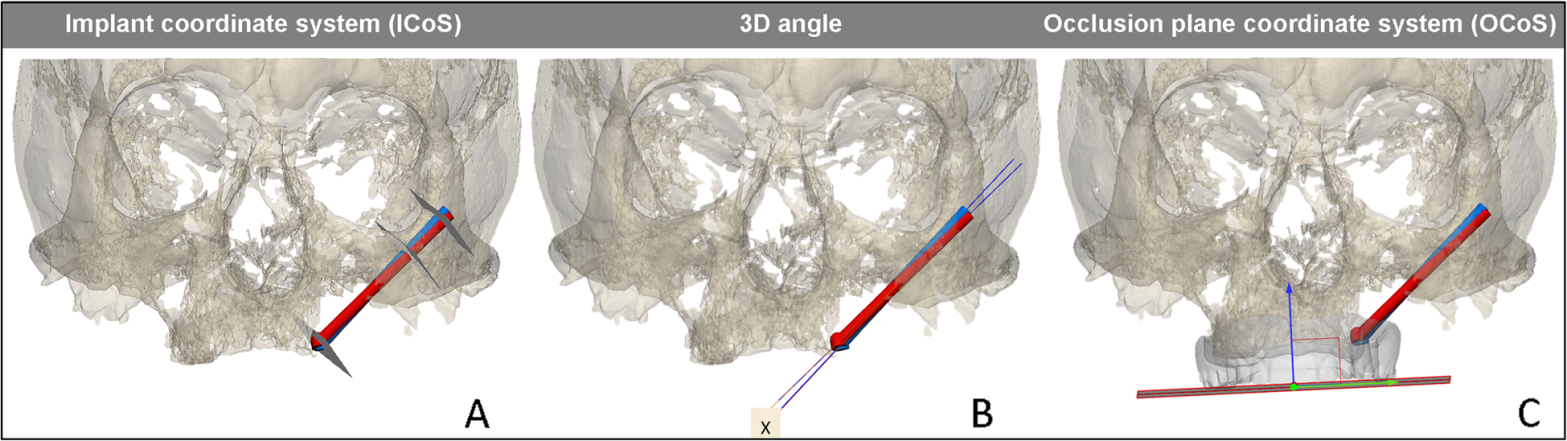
Overview of the several types of measurements and reference planes or coordinate systems for assessing the accuracy of zygomatic implant placement derived from post-op CBCT. In red, the planned zygomatic implant position; in blue, the postoperative zygomatic implant position. Left: the implant coordinate system (ICoS) including the three reproducible reference planes in which the accuracy is measured; the center of the zygomatic implant head, bone entry point of the implant, and bone exit point of the implant. Middle: 3D angular deviation between 3D planned position and post-operative implant position. Right: occlusion plane coordinate system (OCoS). A plane parallel to the prosthetic occlusional plane is defined; perpendicular to this plane is the blue arrow. This arrow indicates the direction in which the abutment height accuracy is calculated
